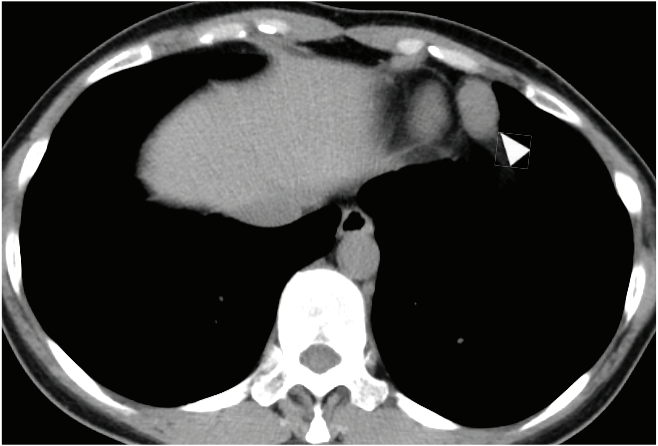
Plain axial CT image showing a solid mass in the left anterior mediastinum (arrowhead).
